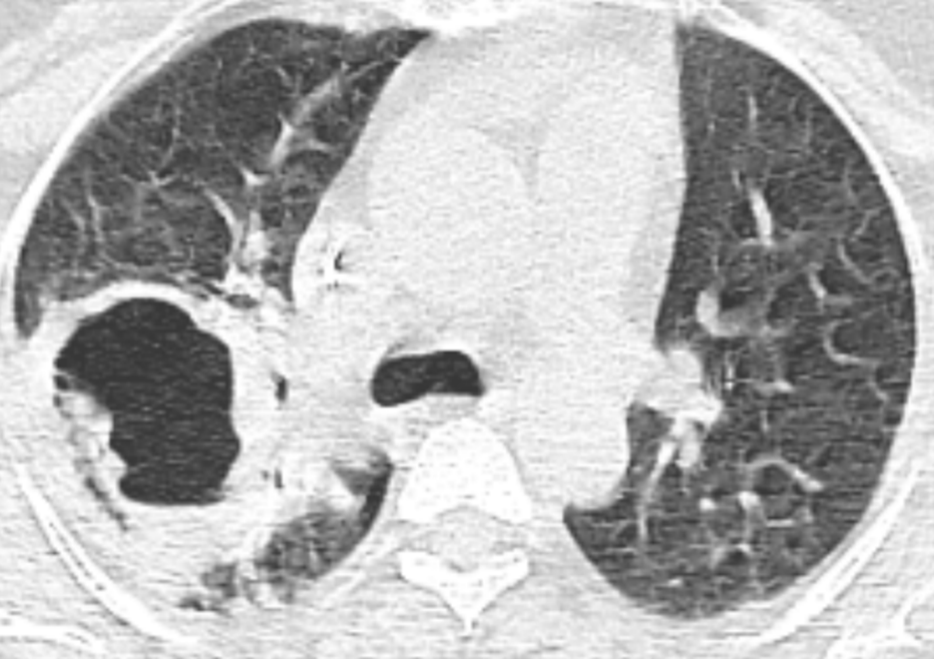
Axial CT chest image in lung window shows necrotic right upper lobe cavity with internal septations and debris on a background of surrounding COVID-19 changes.
